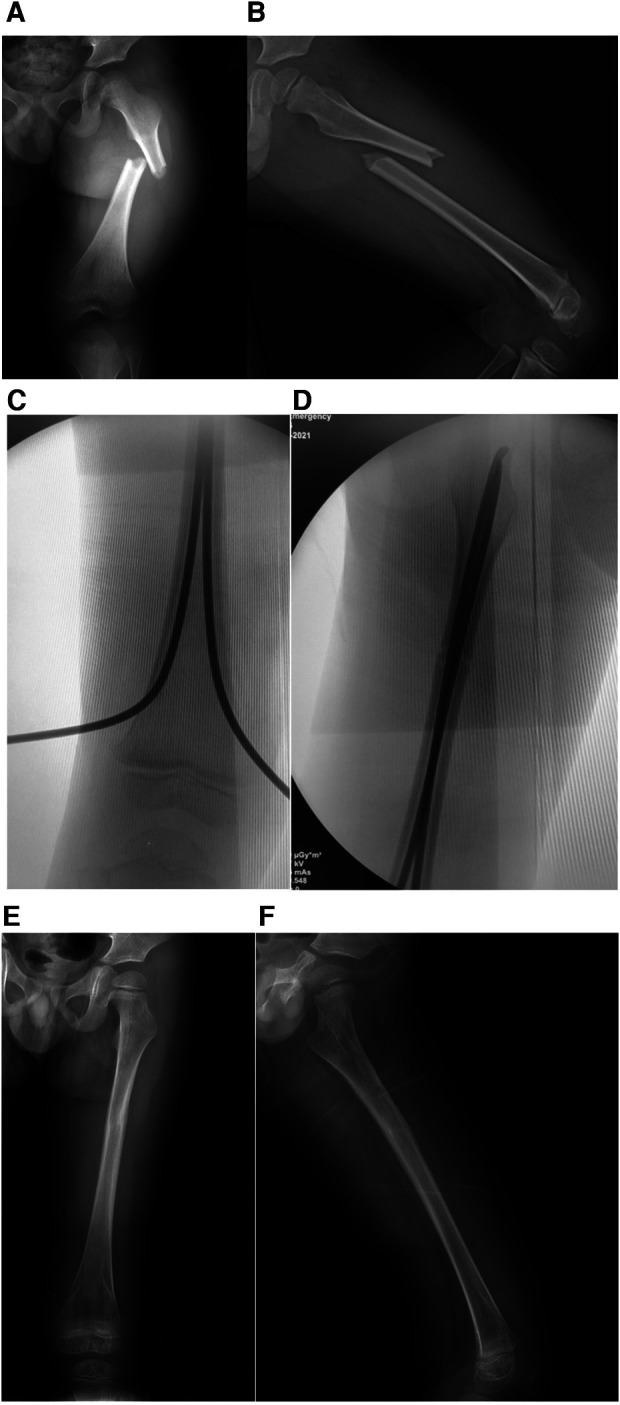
Typical short oblique fracture of left femur of a 4 years old boy. (A) The initial AP x-ray of left femur preoperative. (B) lateral x-ray of left femur preoperative. (C) An entry point was made with the help of a bone awl approximately 2.0–3.0 cm above the physis on the lateral side. (D) C-arm result after closed reduction and ESIN fixation. (E) 10 months follow-up AP x-ray of left femur. (F) 10 months follow-up lateral x-ray of left femur.
